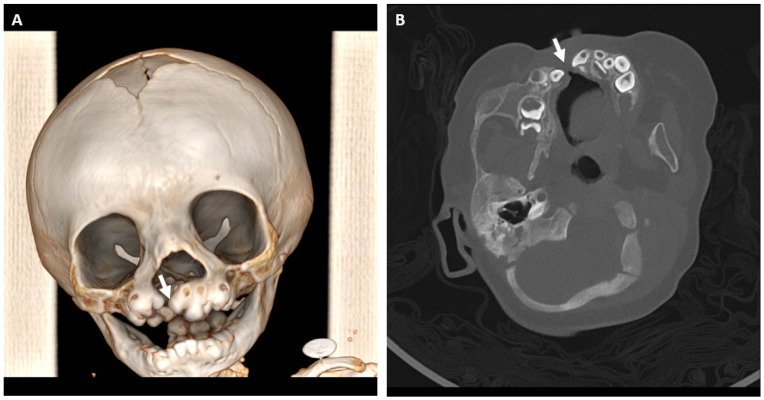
(A) Three-dimensional CT reconstruction of a patient with CHARGE syndrome and cleft lip and palate (arrow). (B) The presence of a cleft through the dental arch is frequently associated with missing and ectopic teeth (arrow).
